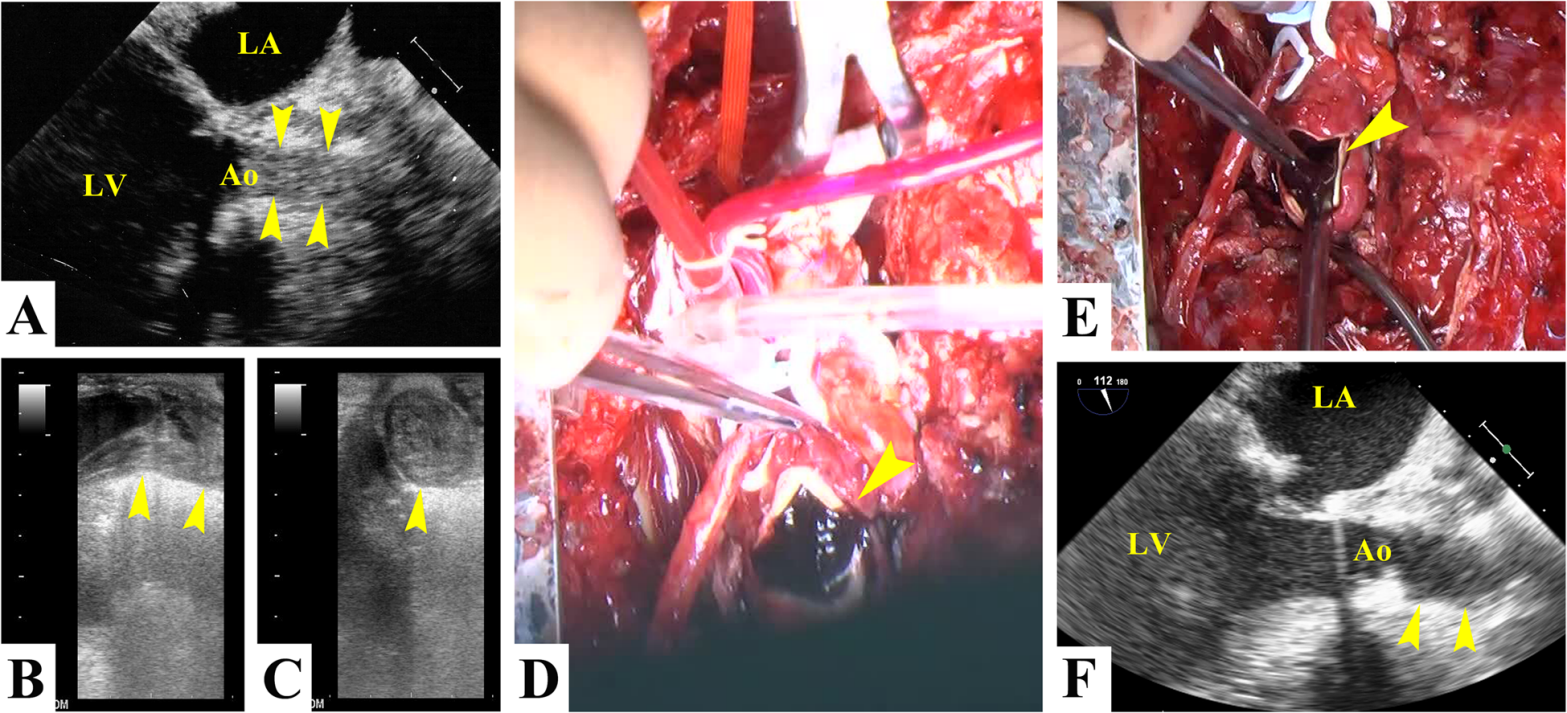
Intraoperative findings. The visualization of the ascending aorta by transesophageal echocardiography was unclear (a, arrowheads). Direct echography in the ascending aorta revealed complete obstruction of the homograft by a thrombus (b, c, d). The thrombus located inside the homograft was removed (e, f)
